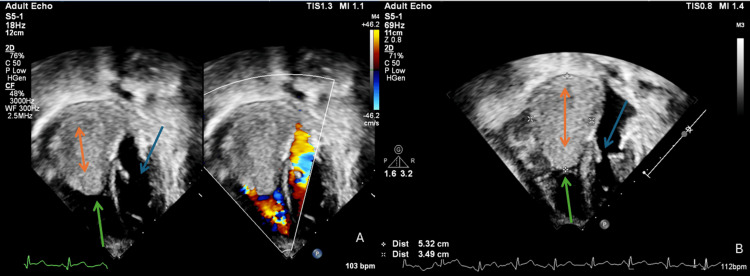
Preoperative four chamber echocardiogram of the heart Preoperative transthoracic echocardiography demonstrating intracardiac tumor extension. (A) Apical four chamber view showing large mass occupying the right atrium and obstructing the tricuspid valve. (B) Measurements of the mass (5.32 x 3.49 cm) as seen from the four chamber view of the echocardiogram. The blue arrow in both figures indicates a clear and unobstructed mitral valve, while the green arrows show an obstructed tricuspid valve, and the orange arrows show the tumor.
